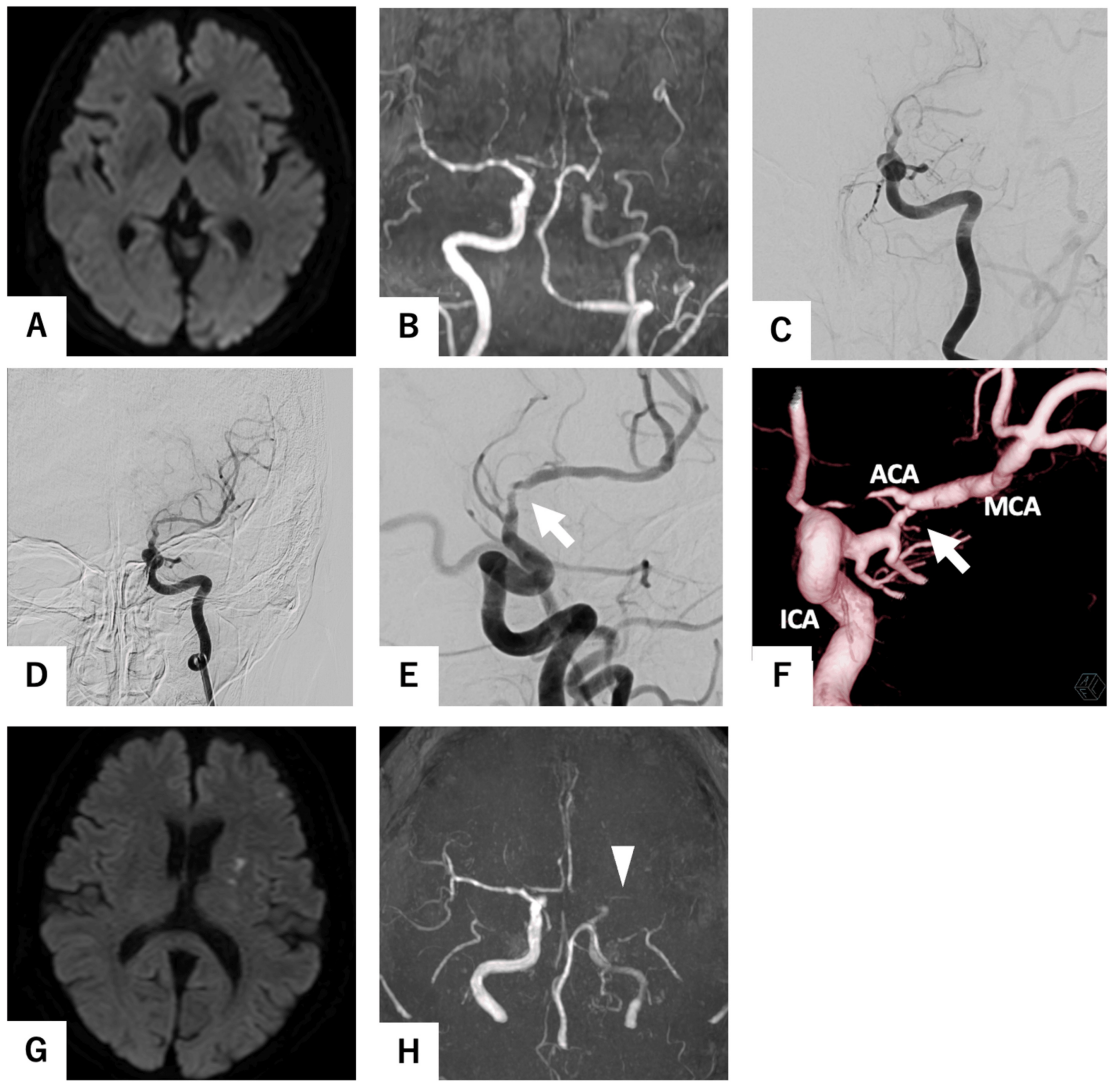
Initial magnetic resonance imaging (MRI), initial cerebral angiography, and MRI on the following day. (A) No high-intensity area was found on the diffusion-weighted image (DWI). (B) Magnetic resonance angiography (MRA) demonstrated an occlusion of the left internal carotid artery (ICA). (C) The initial ICA angiogram revealed a severe stenosis of the left ICA, accompanied by a markedly delayed blood flow to the left middle cerebral artery (MCA) region. (D) The guide catheter was advanced into the ICA and the ICA was spontaneously recanalized. (E) The stenosis remained at the C1 portion (white arrow). (F) A three-dimensional rotational angiogram demonstrated that the stenosis (Warfarin-Aspirin Symptomatic Intracranial Disease (WASID) 55%) was present at the C1 portion (white arrow). (G) The following day, DWI revealed new infarcts in the left basal ganglia and left frontal lobe. (H) MRA revealed a high-signal area in the left MCA (white arrowhead).
